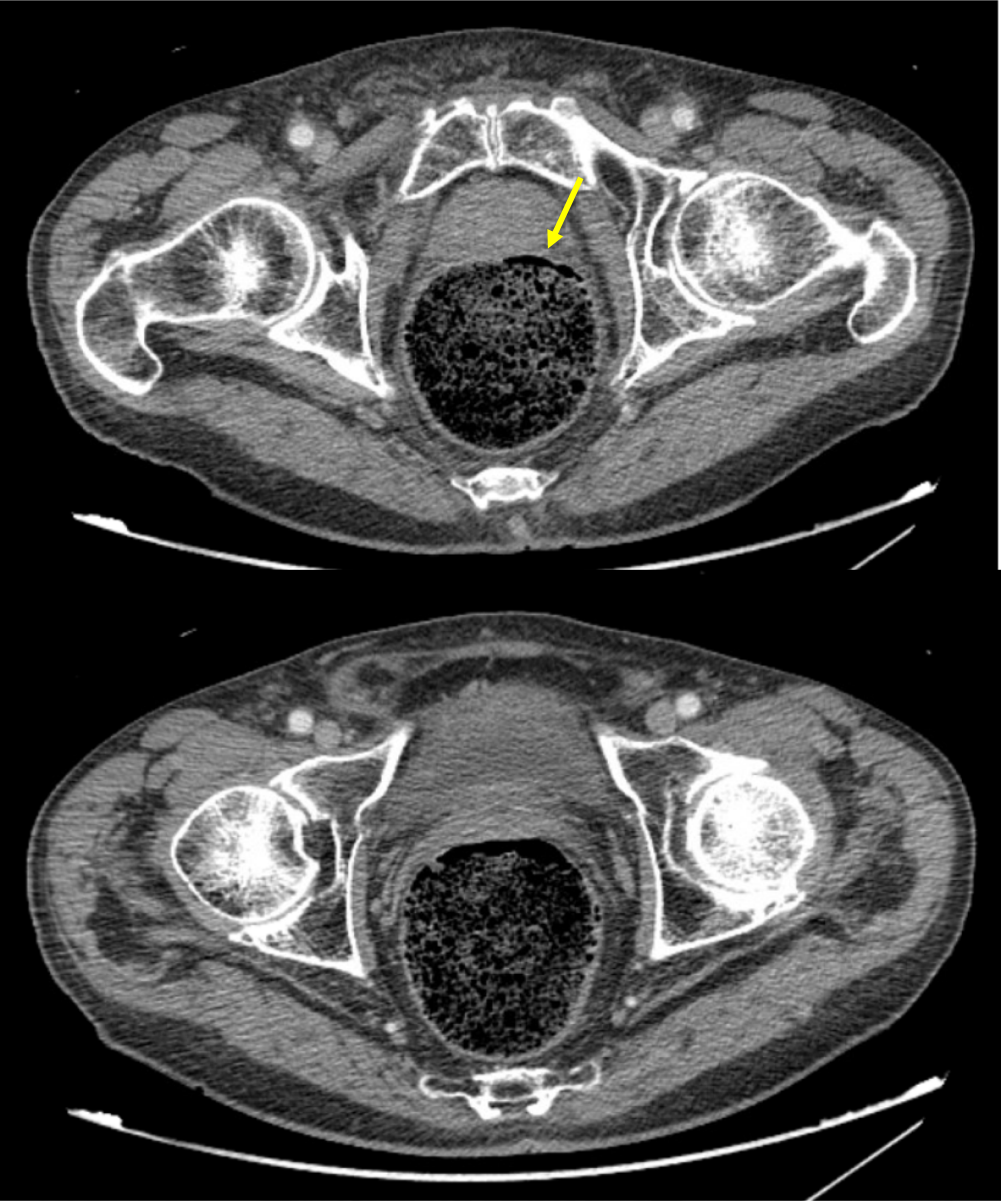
Axial contrast-enhanced CT images of an 89-year-old male with large colonic stool burden, mild rectal wall thickening, and fat stranding, suggesting stercoral colitis. Extraluminal air is seen within the rectal wall or rectal prostatic space, concerning for stercoral ulceration (arrow)
